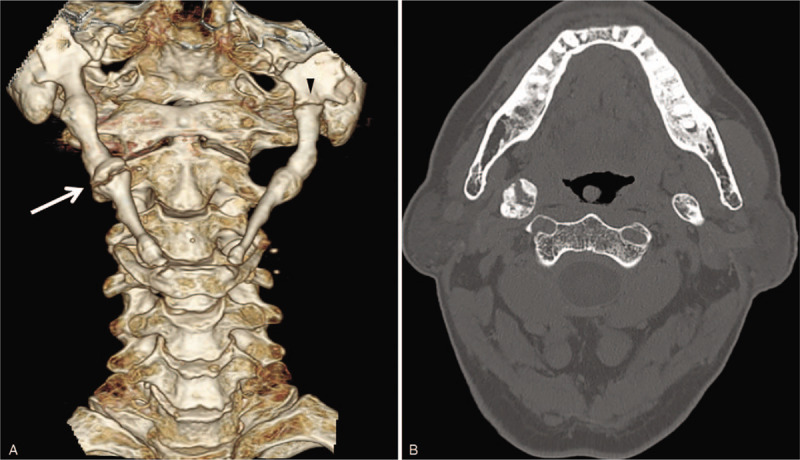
(A) A 3-dimensional computed tomography scan shows bilateral complete stylohyoid complex ossification. A fracture is present on the ampullary area on the right side (arrow) and a gap is seen on the left side of the proximal area (arrowhead). (B) Axial computed tomography demonstrating a fracture on the right ossified stylohyoid ligament.
